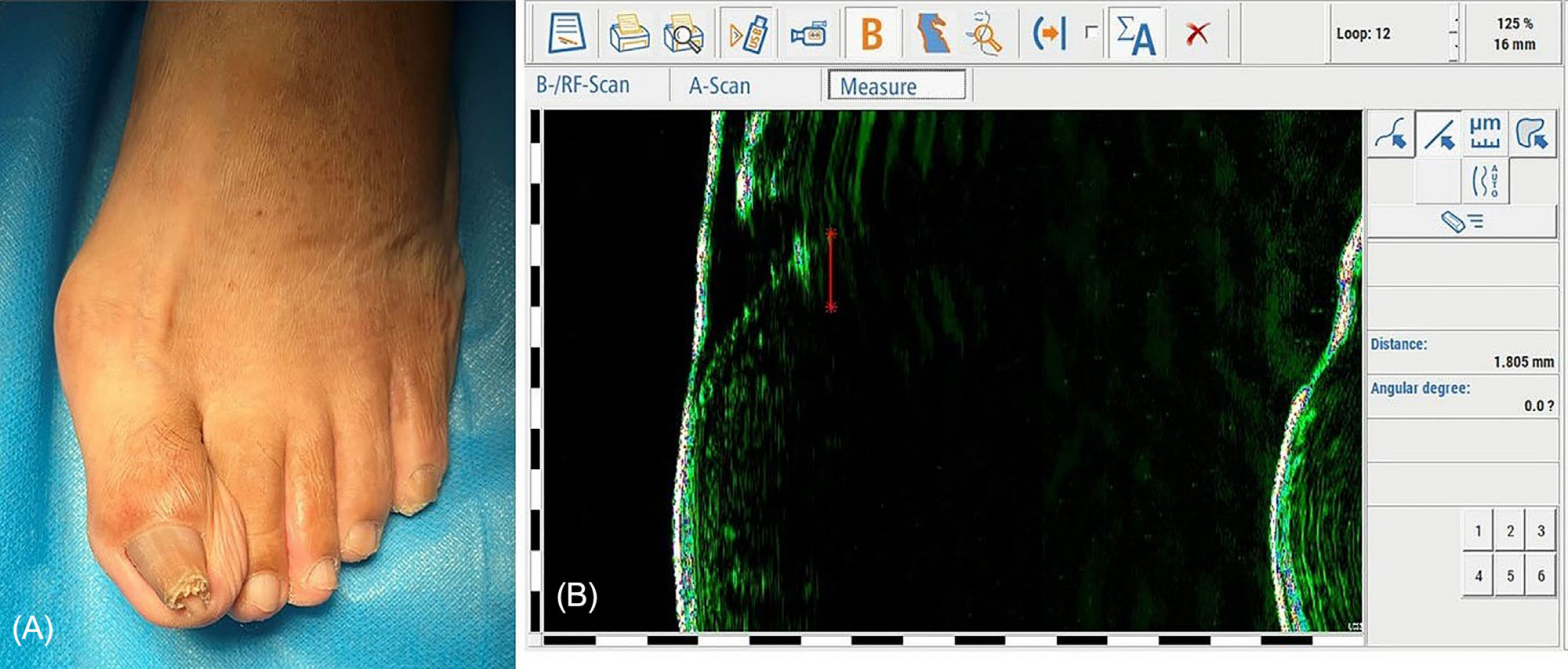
The completely exposed distal nail plate can be measured with the conventional method to obtain height and curvature indexes. The use of high‐frequency ultrasonography (HF‐USG) complemented the data of the covered nail root, which were combined with the apparent width of the nail plate to obtain a more accurate width index. (A) The case of the omega‐type pincer nail. (B) A2 of the HF‐USG width index (1.8 mm) and the width of the nail root buried subcutaneously under HF‐USG (red line).
